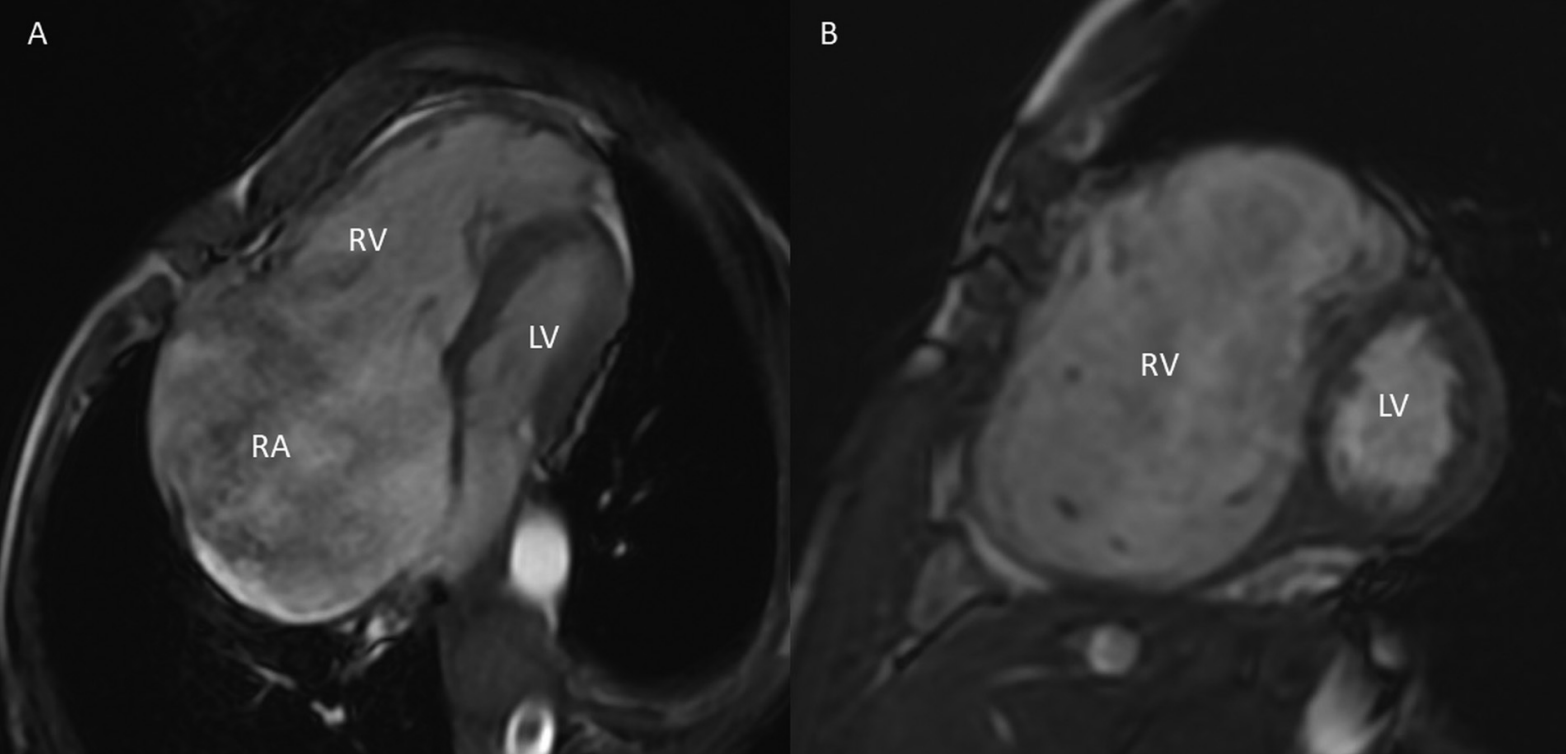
Magnetic resonance imaging showing bright blood sequence in four-chamber view of grossly dilated RA and RV (
